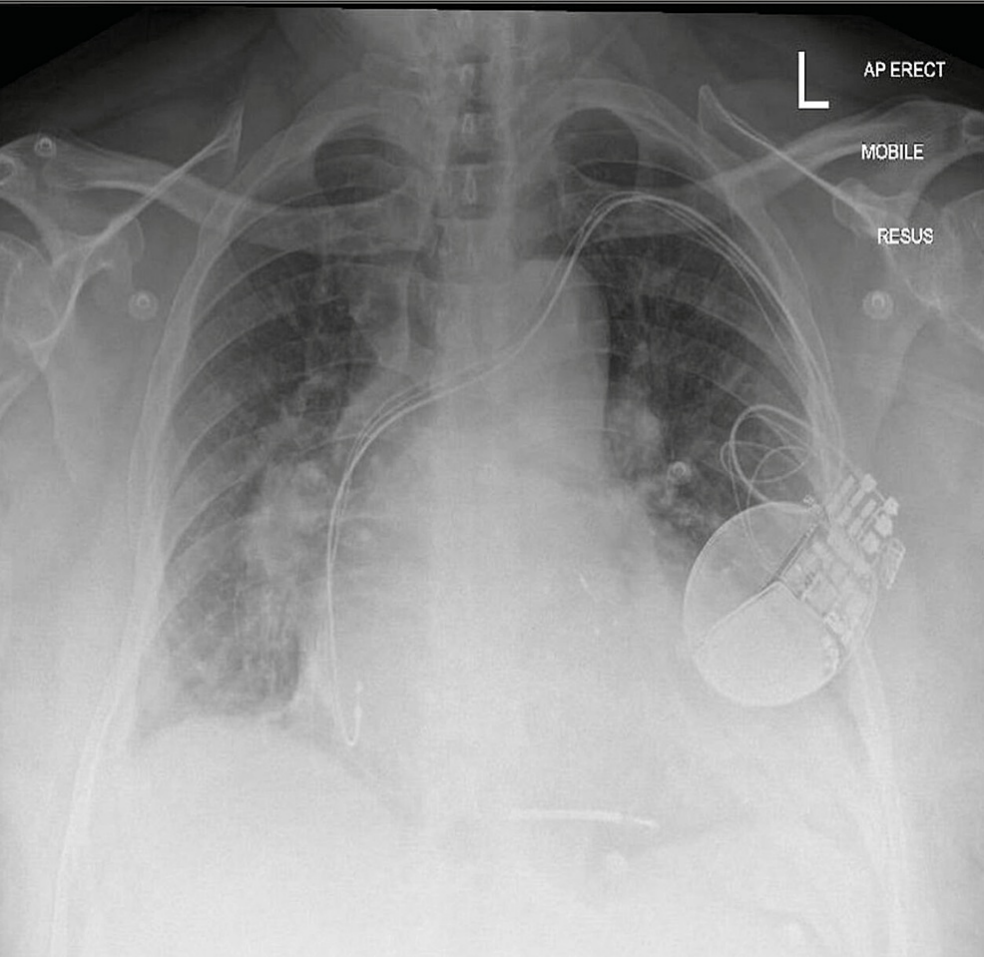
Chest radiography showing pulmonary edema and cardiac resynchronization therapy defibrillator in situ
